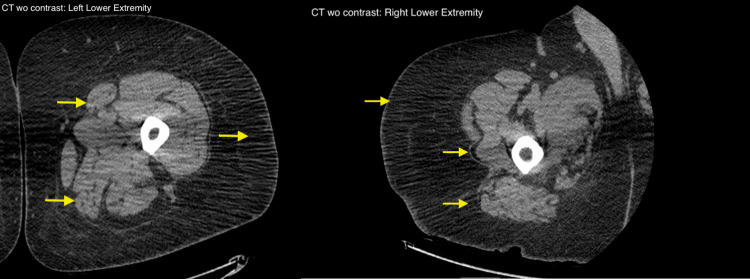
CT without contrast of bilateral lower extremities showing muscular atrophy and subcutaneous edema
